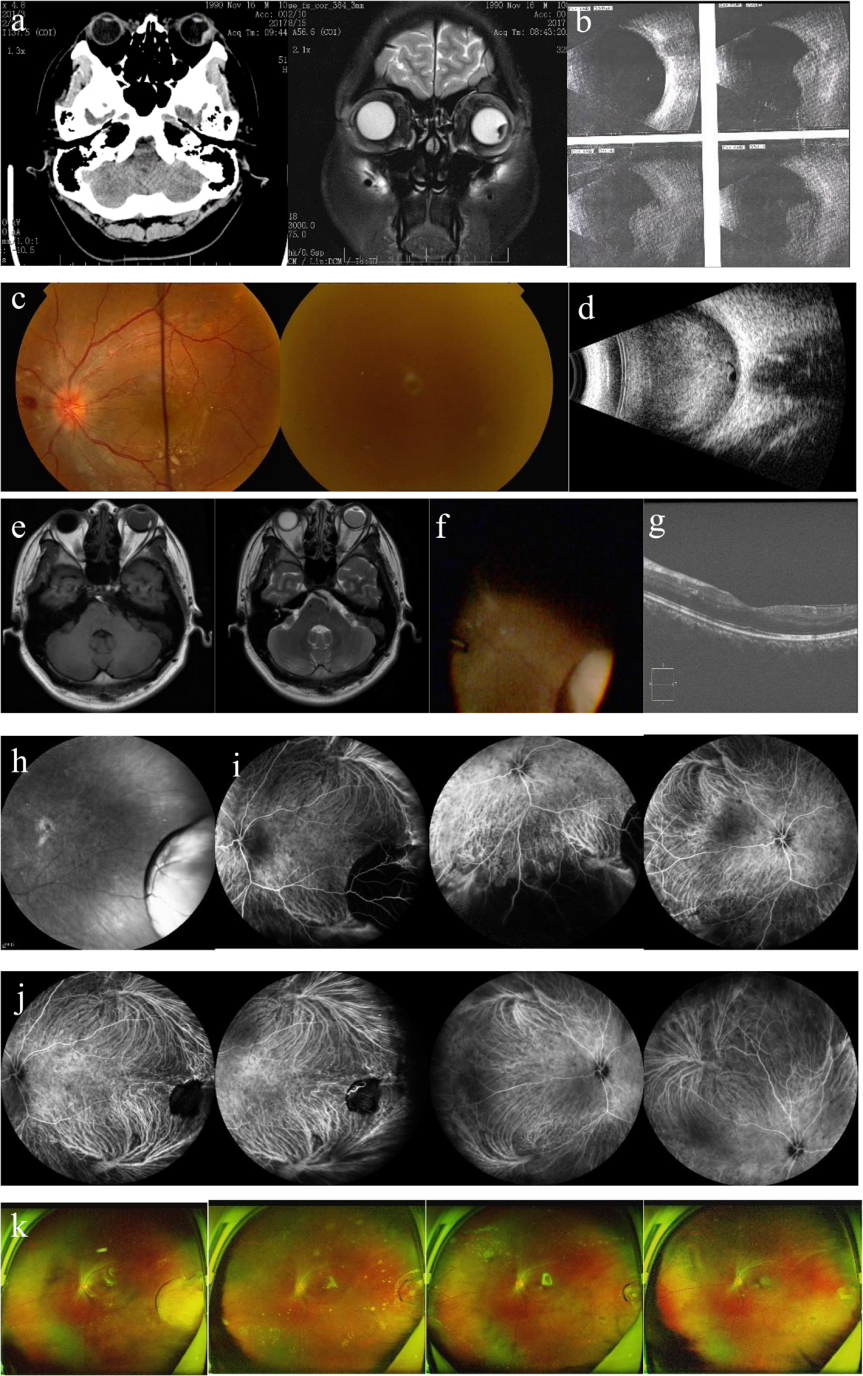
a Skull CT and MRI (other hospital). Abnormal signal shadow in the left eyeball (beside the lateral wall). b B-ultrasonography (other hospital). A dome-shaped retinal detachment of the left eye. c Fundus camera. Disc hemorrhage near the optic disc and yellow and white exudation at posterior pole in the right eye; fundus details could not be evaluated in the left eye because of hemorrhage in the vitreous cavity. d B-ultrasonography. A dense, diffuse intravitreal hemorrhage of the left eye. e Skull MRI. Abnormal signal shadow in the left eyeball (beside the lateral wall), considering the possibility of intraocular hemorrhage. f Intraoperative findings: inferotemporal subretinal focal elevated lesion with clear boundary and yellow color. g Three days OCT after the operation. Any obvious abnormality was not observed in the macular of the left eye. h Three days of infrared photography after the operation. A dome-shaped bulge in the peripheral part of the inferotemporal region of the left eye. i Three days ICGA after the operation. Low fluorescence occlusion and no abnormal fluorescence leakage in the elevated lesion. j Half a year ICGA after the operation. The hypofluorescence occlusion area under the retina of the left eye was significantly smaller than before. k Scanning laser ophthalmoscopy at 3 days, 3 months, 6 months, and 1.5 years after the operation. Subretinal elevated lesion was gradually reabsorbed of the left eye
